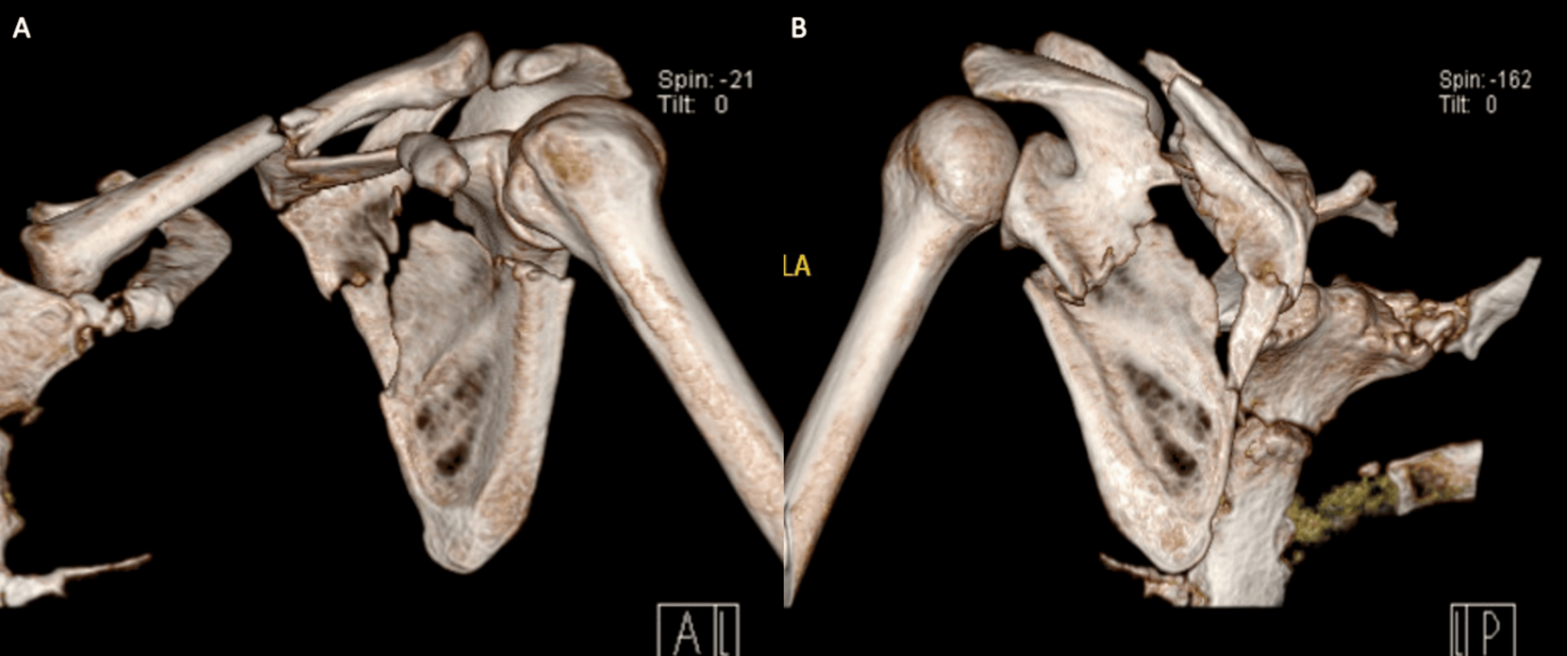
Preoperative 3D CT A: 3D CT of left scapula (anterior aspect)
